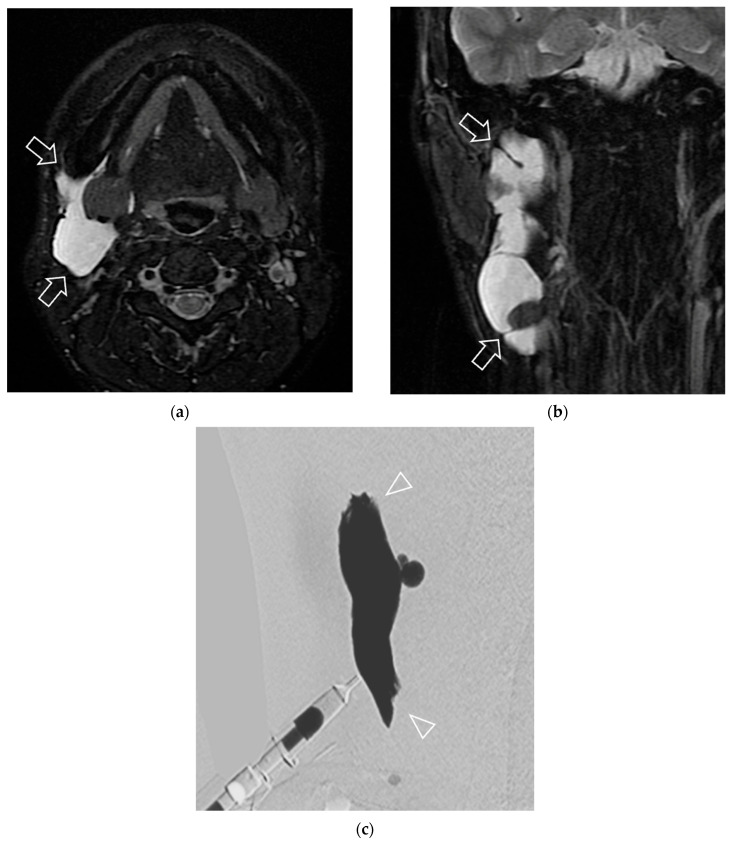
A 21-year-old patient presenting with a palpable mass in the right neck with response to sclerotherapy. (a) A pretreatment axial T2-weighted image with fat suppression (b) A pretreatment coronal T2-weighted image with fat suppression revealed a lobulated macrocystic lesion with high T2 signal intensity within the right submandibular space or deep-seated lesion (arrows). (c) Pattern of contrast opacification on frontal projection of dynamic digital radiographic images corresponding to image b exhibited cavitary morphology (arrowheads). Aspiration of the lesion yielded clear lymphatic fluid. After undergoing one session of bleomycin injection, he experienced improvement in symptoms, with a significant 70% reduction in size confirmed by physical examination and MRI.
